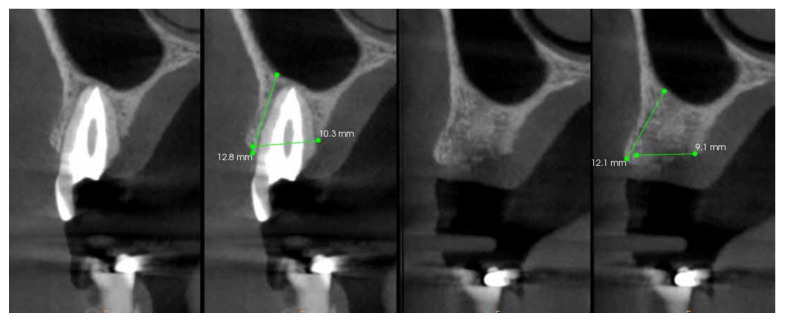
Measurements performed on the CBCT0 and CBCT1: alveolar bone width (ABW) and alveolar bone height (ABH).
